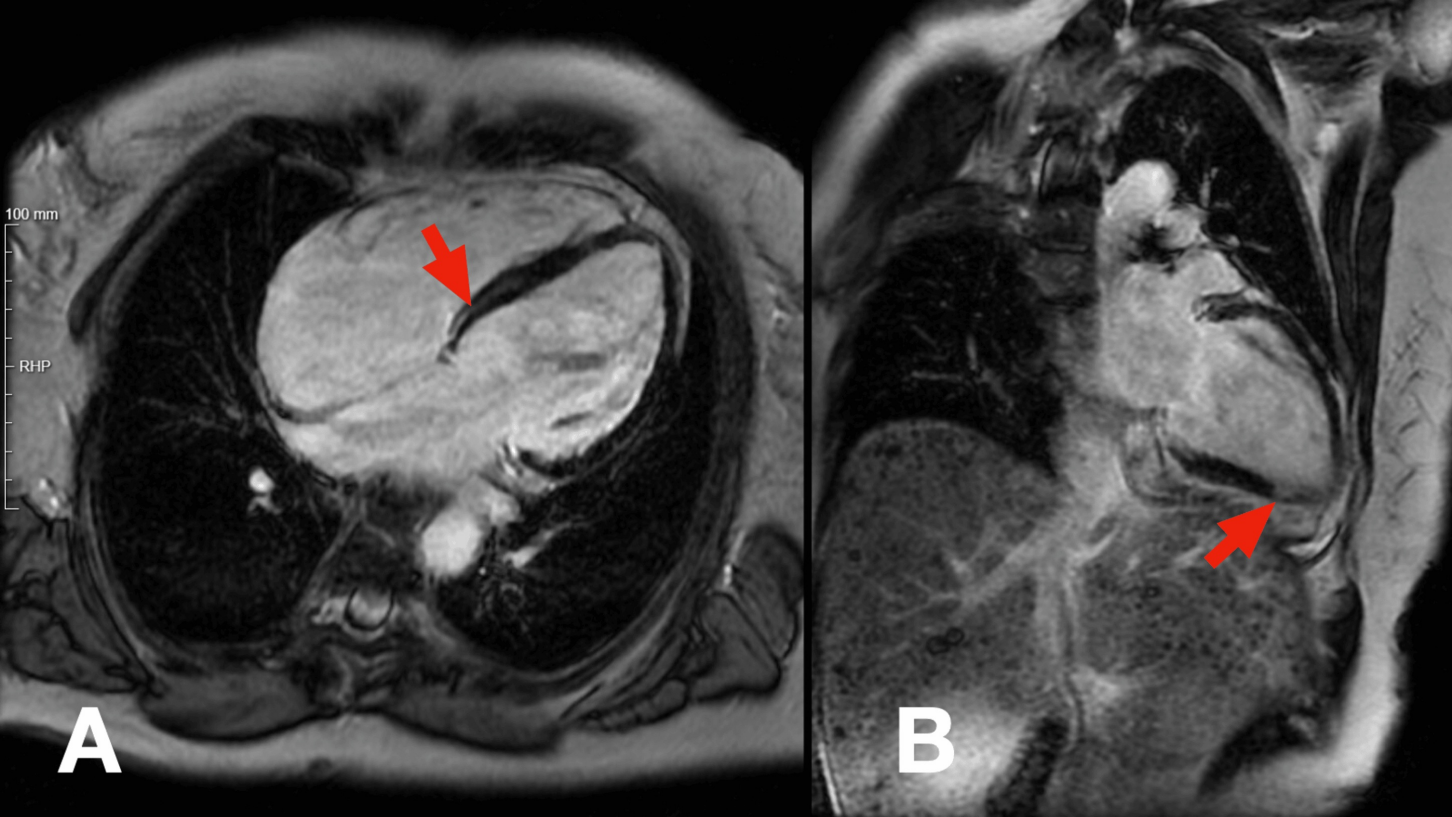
Cardiac MRI with late gadolinium enhancement Red arrows in panels A and B show moderate myocardial fibrosis in a mixed pattern, involving both the subendocardial and mid-wall regions.
